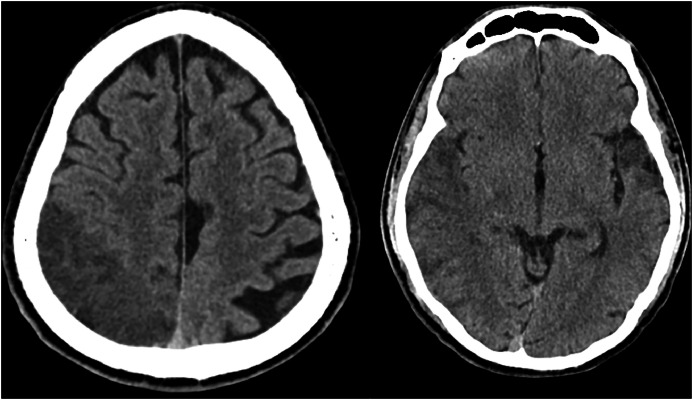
Non-contrast head CT shows loss of gray-white matter differentiation and sulcal effacement in the right MCA and posterior watershed territories, consistent with cytotoxic edema.
